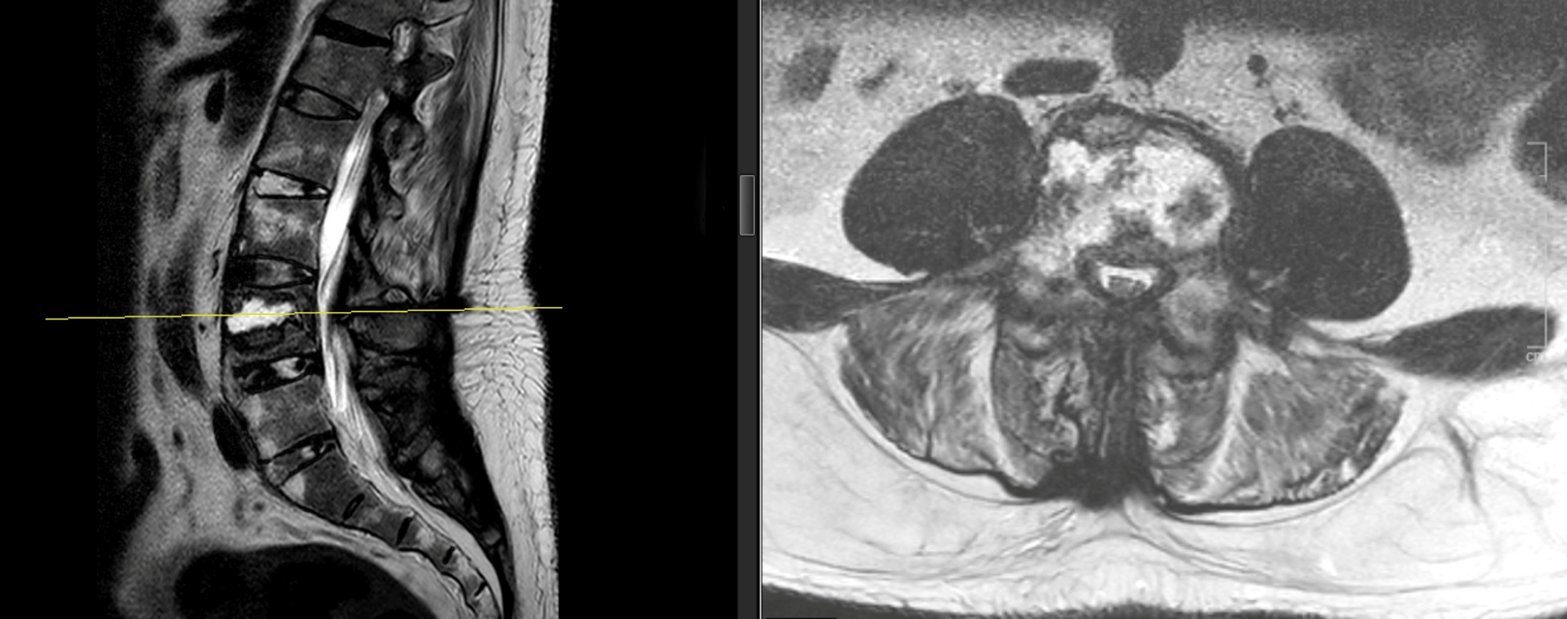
T2-weighted image shows L4 vertebra chance fracture causing the abutment of bilateral L4 traversing nerve roots and possibly the impingement of left L4 exiting nerve root
